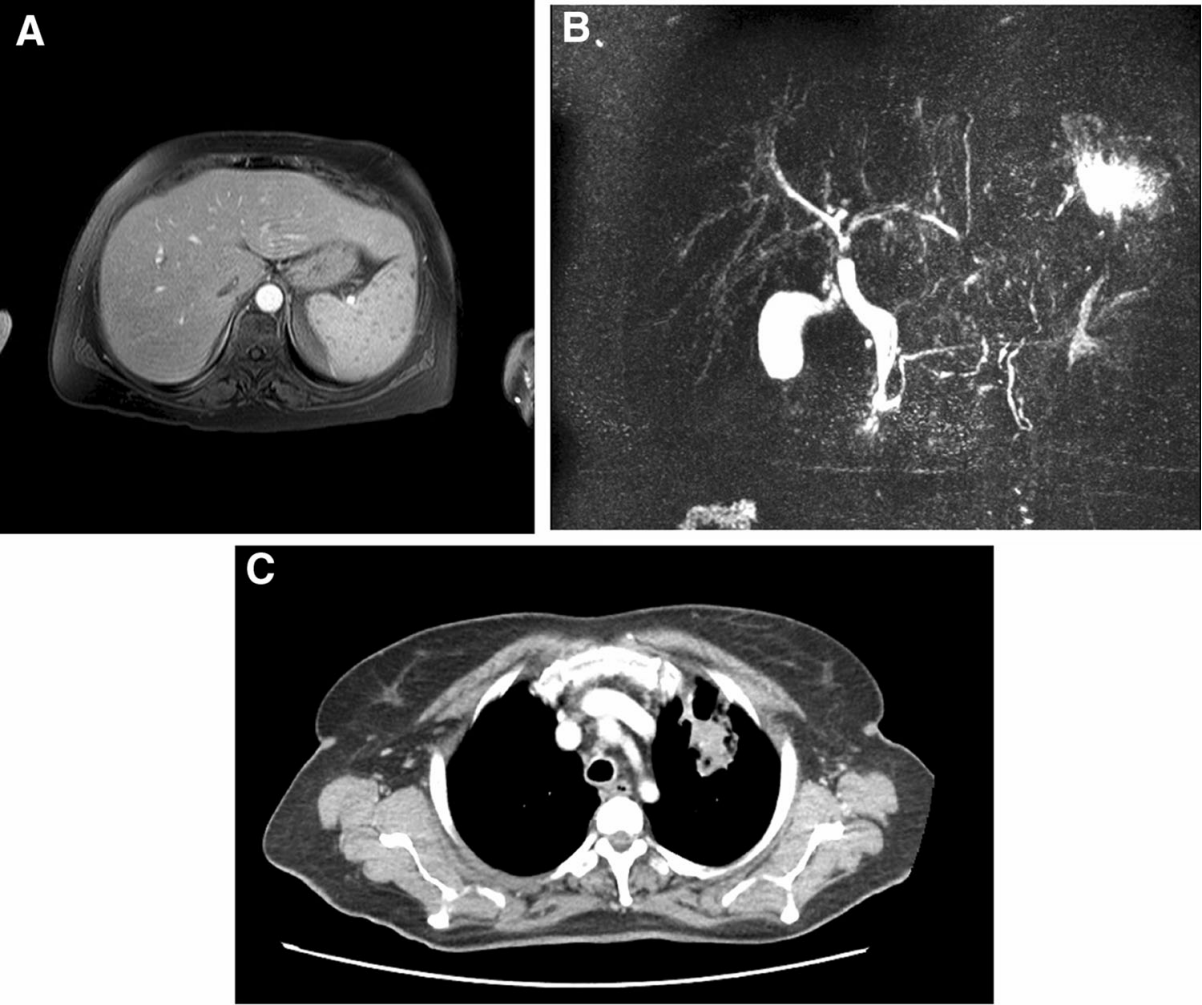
The magnetic resonance imaging shows multiple splenic lesions (a) and reduction of caliber of the proximal extrahepatic bile duct with ectasia of bile duct downstream (b) at disease onset. One month later, a new total body computerized tomography describes a dense tissue (diameter of 3 cm) with irregular margins infiltrating the upper lobe of the left lung, associated with ipsilateral hilar-mediastinal lymphadenopathy (c)
